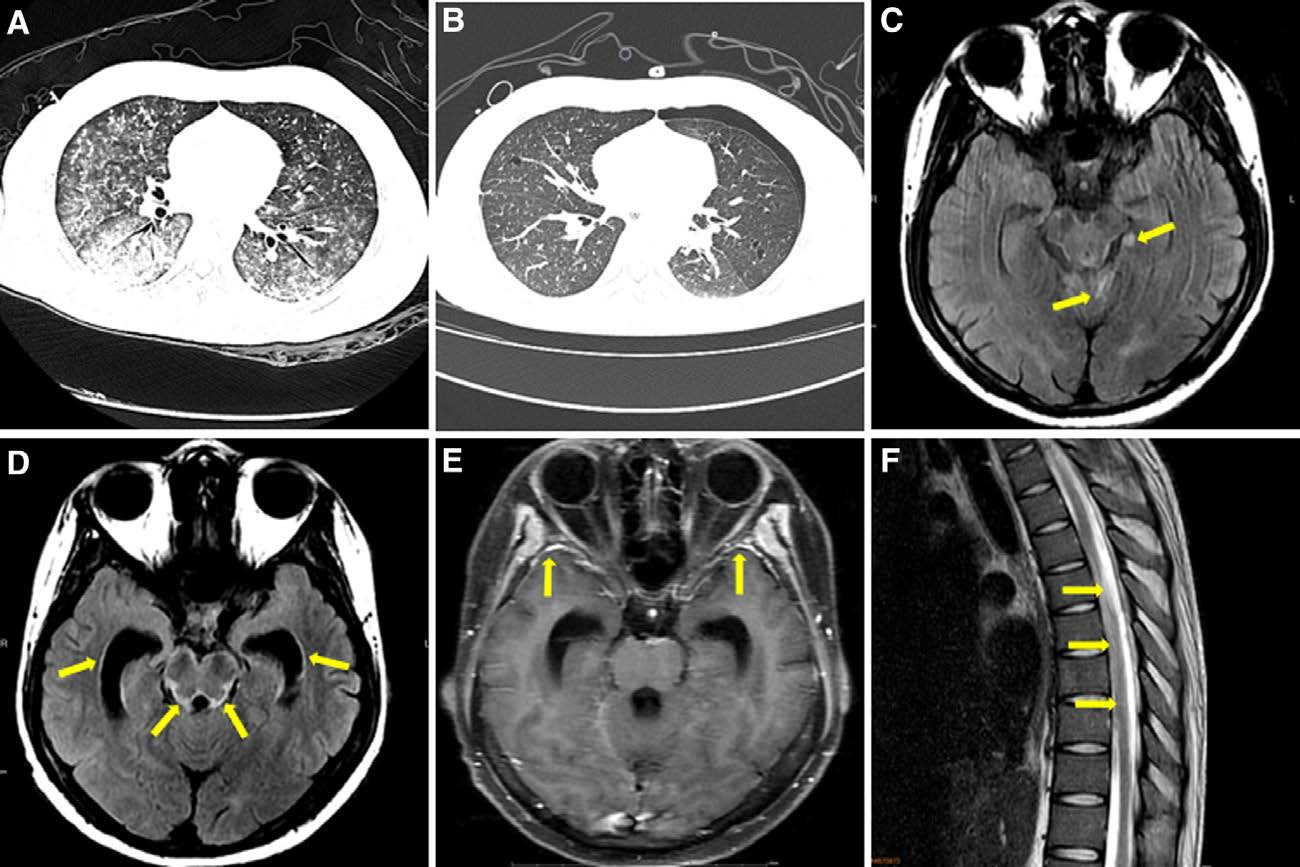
Imaging examination of the patient. (A) The chest computed tomography (CT) scan showed bilateral pulmonary interstitial infiltrates on 1 June 2022, (B) the follow-up chest CT scan showed improvement in the pulmonary lesions on 25 July 2022, (C) the T2WI FLAIR showed high signals in the left parahippocampal gyrus and cerebellar vermis on 1 June 2022 (yellow arrow), (D) the T2WI FLAIR showed widening of the ventricle on 21 July 2022 (yellow arrow), (E) the contrast-enhanced T1WI showed meningeal enhancement on 21 July 2022 (yellow arrow), and (F) the T2WI FLAIR showed longitudinally extensive spinal cord lesions on 21 July 2022 (yellow arrow).
